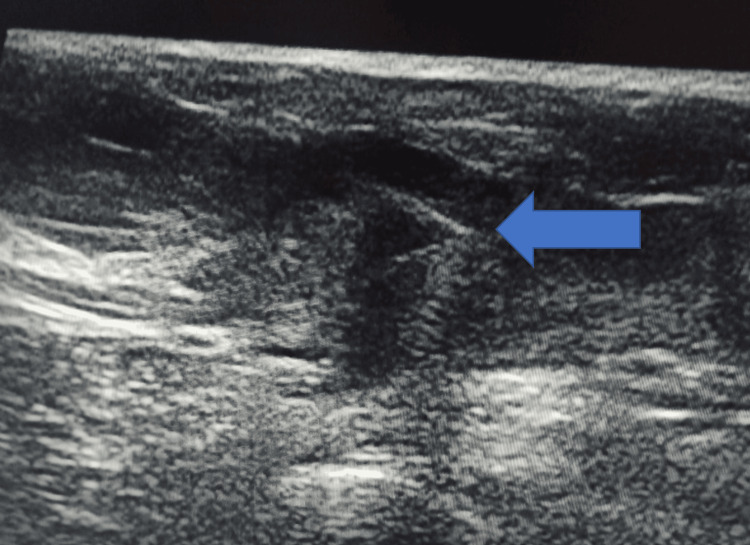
The post-chemotherapy image shows the reduction in size of the tumor that became impalpable clinically, and the marker led to surface marking of the lesion under ultrasound guidance, and the surgeons did the lumpectomy of the patient
